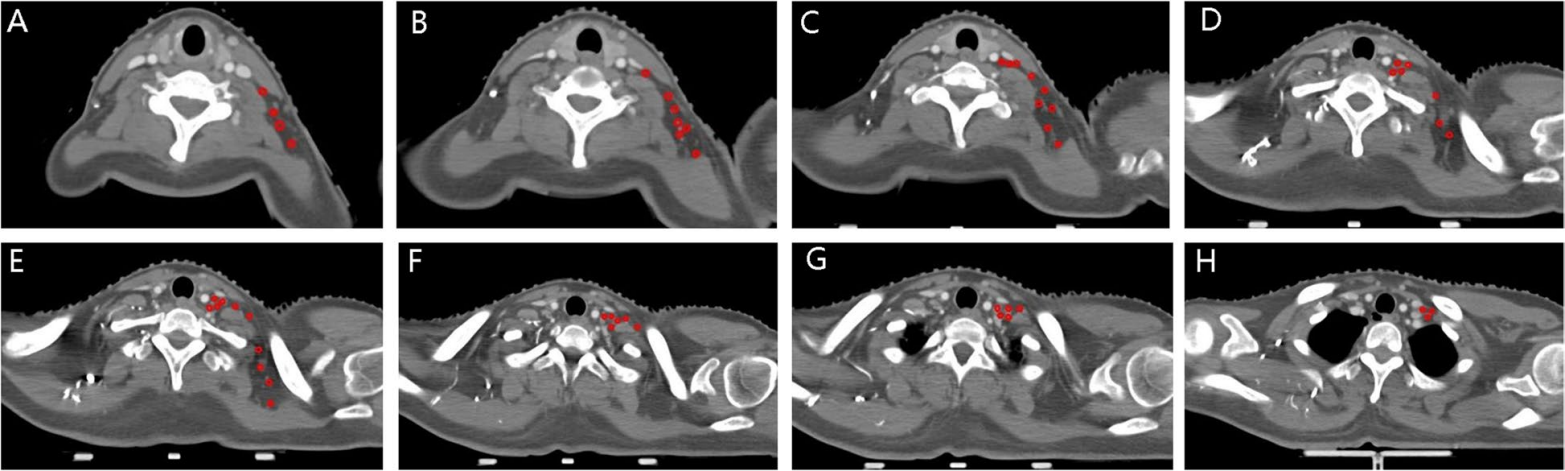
A-H The centre of positive supraclavicular lymph nodes were identified and projected into the template CT images with red circles of 4 mm diameter
